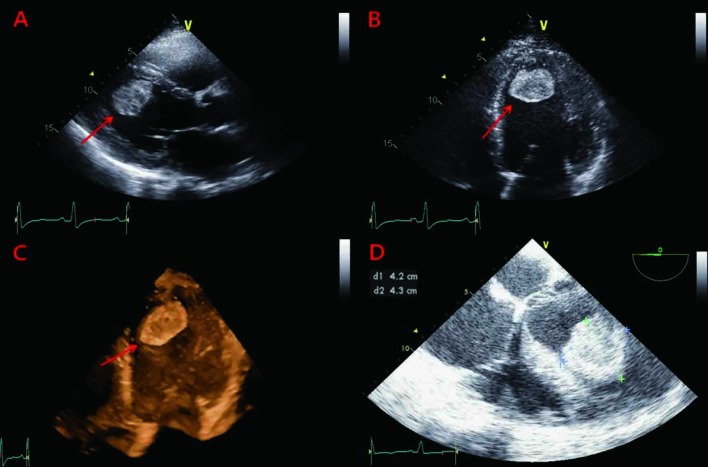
Transthoracic (A-C) and transesophageal (D) echocardiograms showed severe global LV systolic dysfunction and dilatation with a large mobile pedunculated mass in the LV apex.
